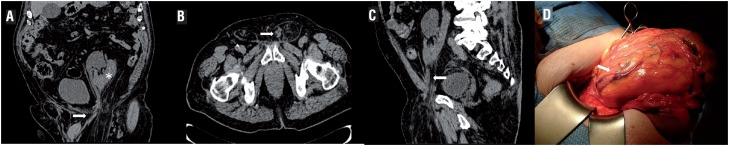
A) Coronal abdominal non-contrast-enhanced CT scan showing dilated left pelvic kidney (asterisk) and dilated ureter (arrow) in the inguinal hernia. B) Axial non-contrast-enhanced CT scan with loop of the left ureter in the hernia (arrow). C) Sagittal non-contrast-enhanced CT scan with ureteral inguinoscrotal hernia (arrow). D) Identification of left ureter (arrow) during the inguinal hernia repair.
